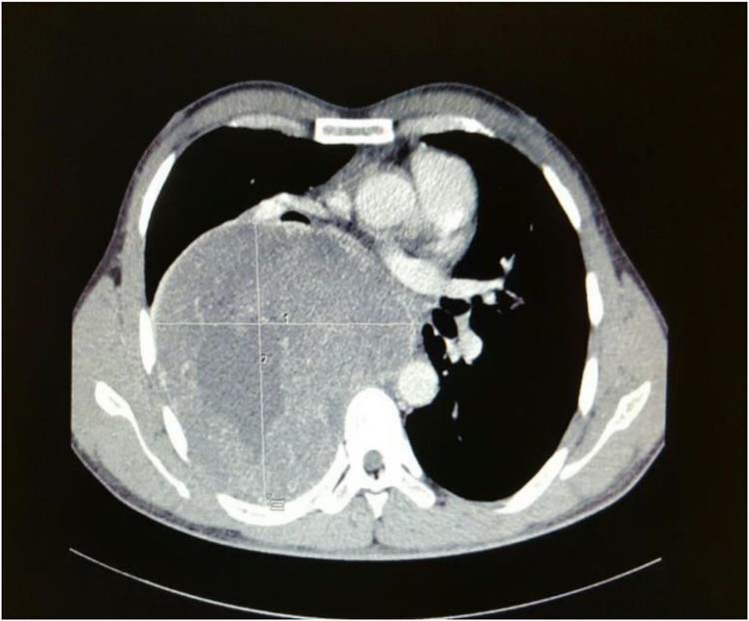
Axial cut of a contrasted CT Thorax depicting a large right posterior mediastinal mass.
